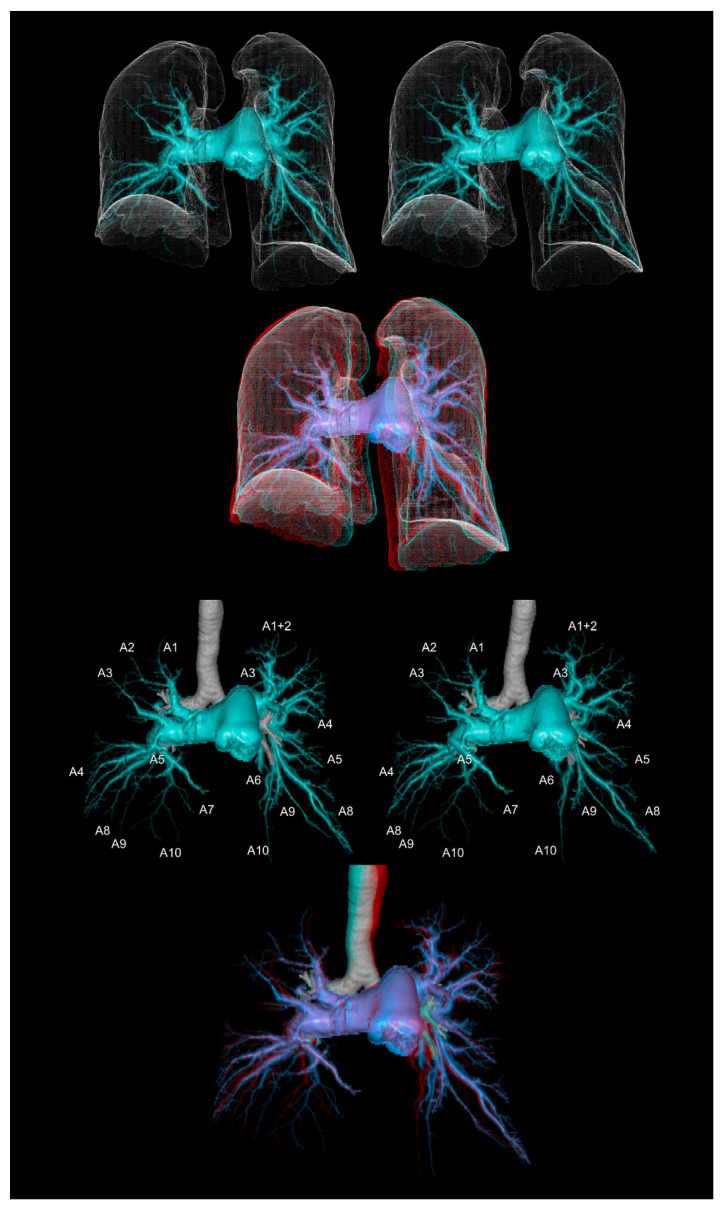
The computed tomographic pulmonary angiography of a patient with chronic thromboembolic pulmonary hypertension. The upper panels show the pulmonary arteries with the lung contours. The lower panels show the pulmonary arteries with the trachea and main bronchi. The first and second panels are aligned for cross-eyed viewing. The third panel is the anaglyph. To see the anaglyph, anaglyphic glasses (red/cyan) are required.
